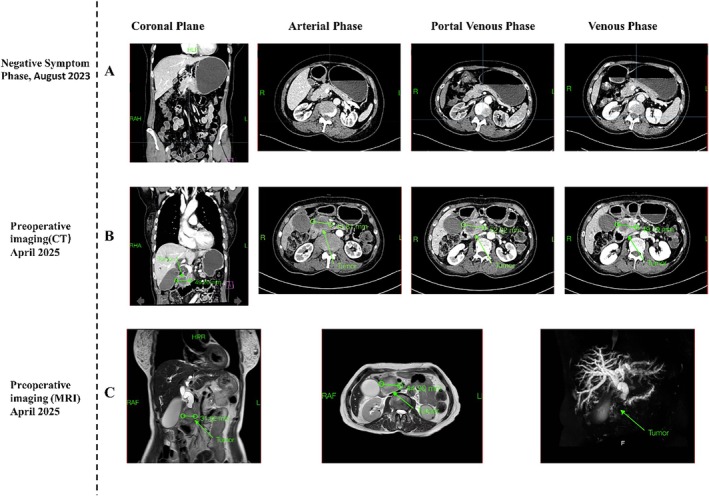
Disease progression of the patient. (A) In August 2023, contrast‐enhanced CT showed no abnormalities in the liver and pancreas. (B) In April 2025, preoperative contrast‐enhanced CT demonstrated a 4.0 × 5.0 cm mass in the pancreatic head with central non‐enhancing necrosis and peripheral ring enhancement, accompanied by gallbladder distension (Red arrows), but no hepatic lesions. (C) The lesion shows hyperintensity on T2‐weighted images with slightly irregular walls, no *liver metastasis*, bile ducts and the pancreatic duct dilation. Red arrows indicate a 4.8 × 4.8 cm tumor.
